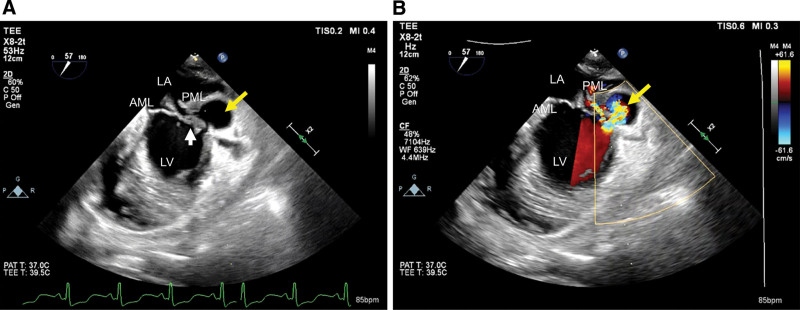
A. Transesophageal cardiac ultrasonography shows the pseudoaneurysm (yellow arrow). The vegetation on the posterior mitral leaflet (white arrow) is shown. B. Transesophageal cardiac ultrasonography image shows the pseudoaneurysm (yellow arrow). The vegetation on the posterior mitral leaflet (white arrow) and the shunt (yellow arrow) are indicated. AML = anterior mitral leaflet, LA = left atrium, LV = left ventricle, PML = posterior mitral leaflet.
